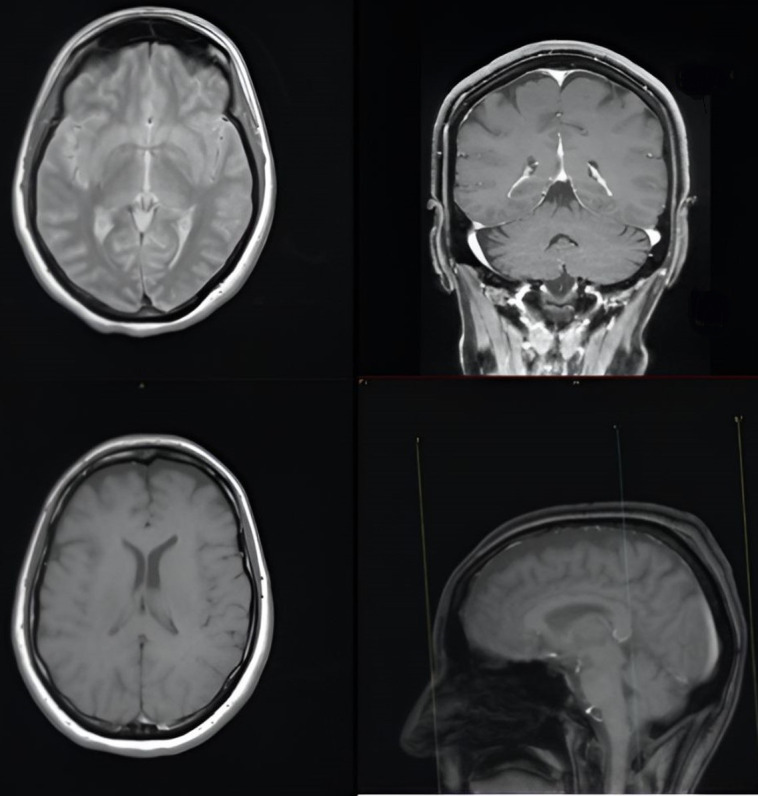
Brain magnetic resonance imaging with contrast.
